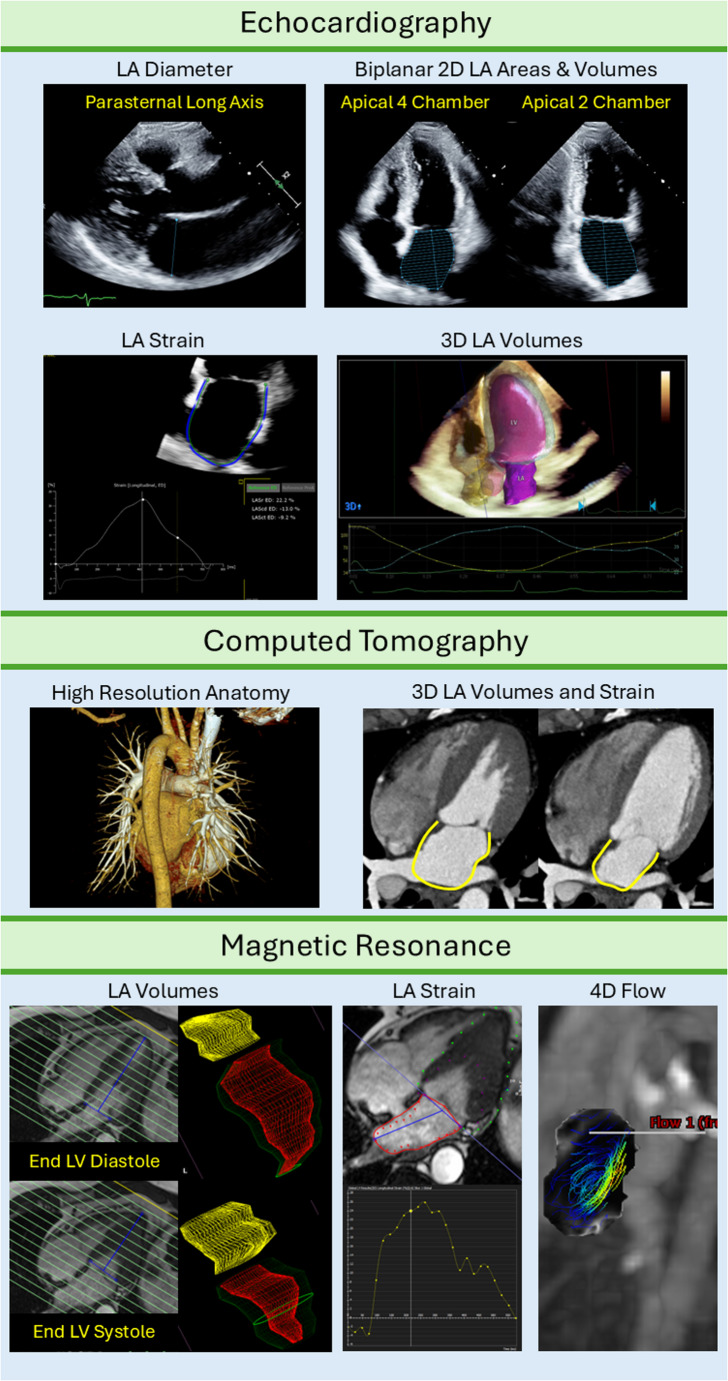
Examples of Multimodality Imaging Assessment of Left Atrial Function. Examples from multimodality imaging of LA size and function. Echocardiography allows for linear, 2D, and 3D measurements, including volumetric- and strain-based functional evaluation. Computed tomography provides high resolution anatomy visualization, also with the capacity for volumetric and strain assessments. Magnetic resonance imaging can similarly provide high resolution imaging of the LA for volumetric and strain-based characterization, as well as complex flow computations for calculating measures of stasis. LA— left atrial; LV— left ventricular
